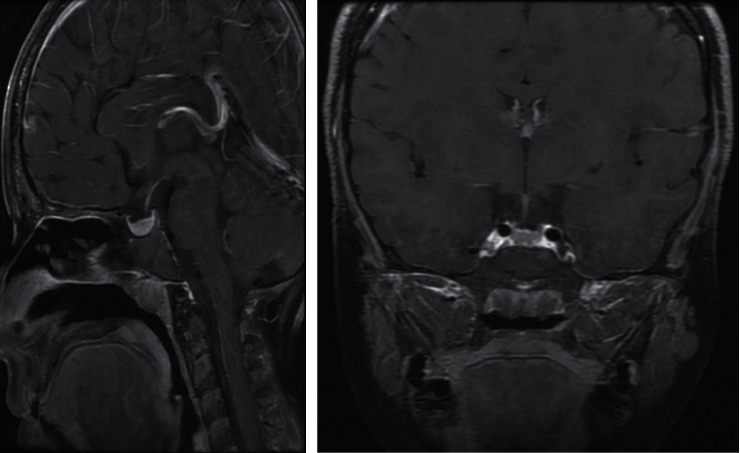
Sagittal and coronal contrast-enhanced T1-weighted magnetic resonance images taken at follow-up after 6 months of thyroid hormone replacement therapy. The images showed a moderate interval reduction in the size of the pituitary mass.
